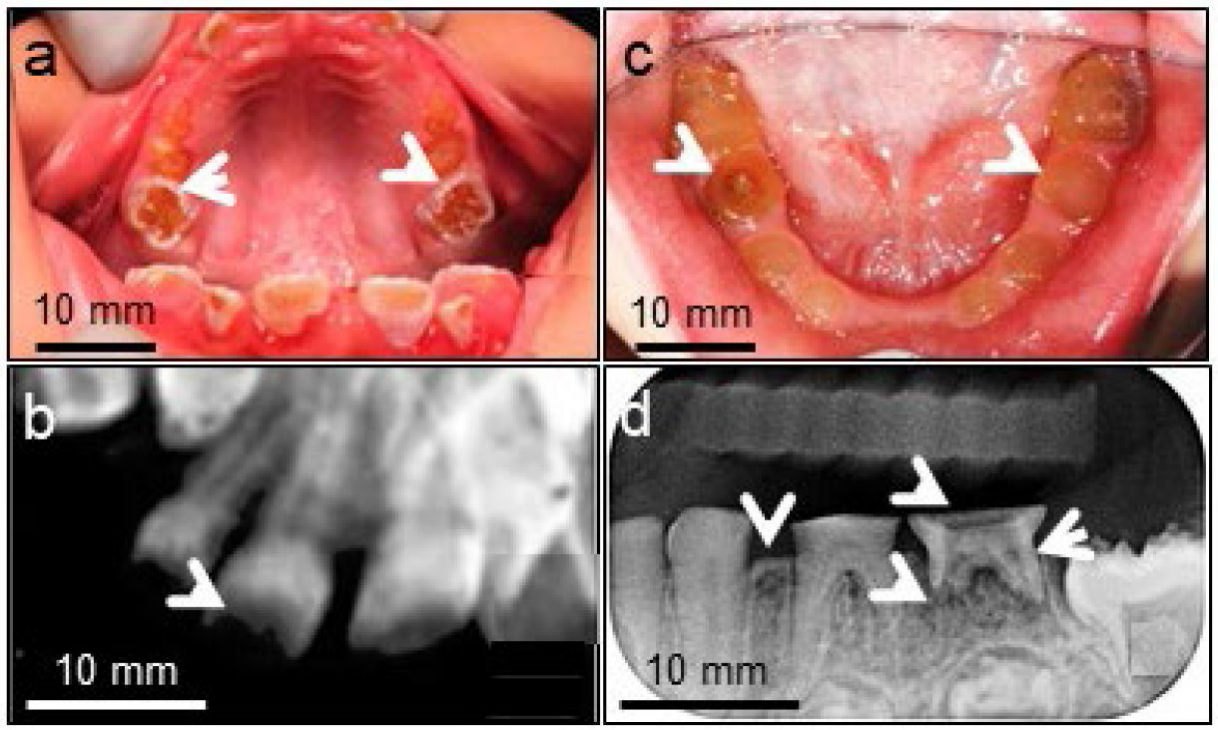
Clinical photographs and radiographs from DGI-II patients. Clinical photograph of a 7 year-old boy showing (a) severe attritions (arrowheads). (b) Radiograph indicates severe enamel loss with decreased pulp space and reduced dental mineral density. (c) Intraoral photographs of a 5 year-old girl exhibiting severe attrition of the primary dentition to the gingiva level and teeth with yellow-brown colour and a translucent appearance (arrowheads). (d) Radiograph shows that dentine was thin, with severe occlusal attrition and periapical abscess (arrowheads).
